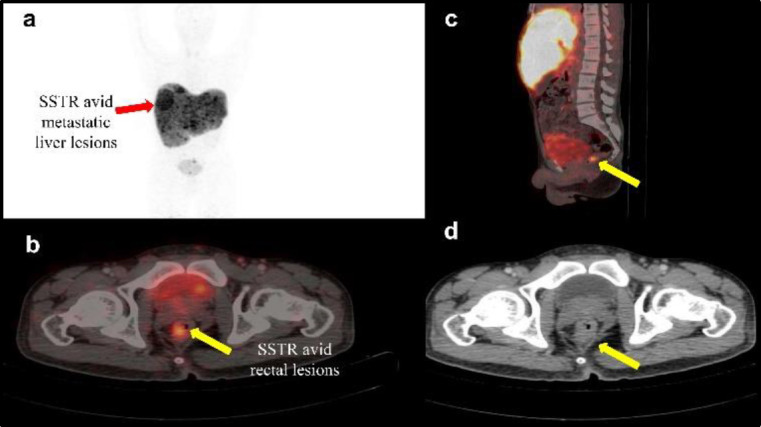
[^68^Ga]Ga-DOTATATE PET/CT Scan showing uptake in: (a) SSTR-avid metastatic liver lesions, (b) SSTR-avid rectal lesion, (c) SSTR-avid rectal lesion: sagittal fused image and (d) axial CT image
